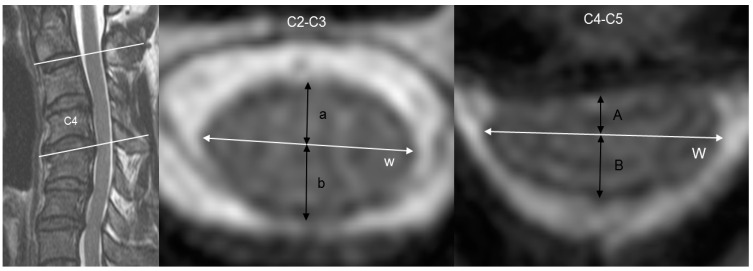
Cervical magnetic resonance images of a 49-year-old male patient with cervical spondylotic myelopathy and kyphotic alignment. He complained of severe hand clumsiness and whole-body numbness. His total Japanese Orthopaedic Association (JOA) score was 12 (I-0, II-4, III-5 and IV-3, respectively). The measured values were as follows: 3.7 mm (a), 3.9 mm (b), 12.9 mm (w), 1.8 mm (A), 3.7 mm (B) and 14.6 mm, respectively (W). The anterior length of the spinal cord decreased from 3.7 mm to 1.8 mm, but the posterior length slightly decreased from 3.9 mm to 3.7 mm.
